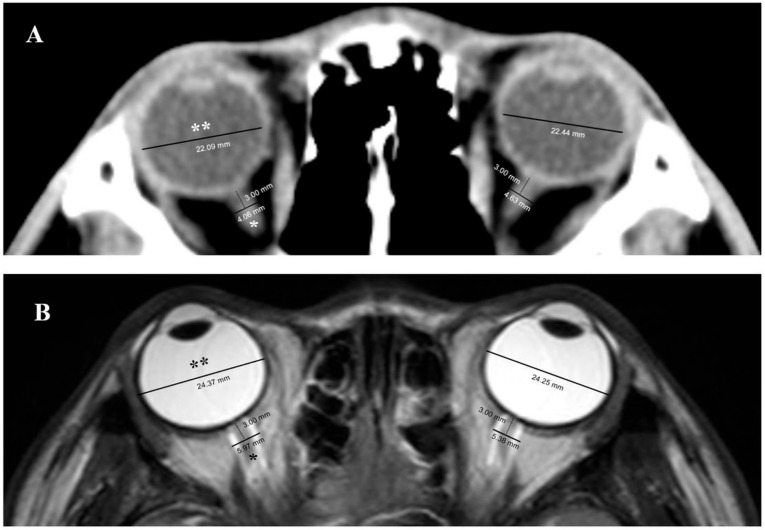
Measurement of the optic nerve sheath diameter and eyeball transverse diameter using computed tomography (A) and magnetic resonance imaging (B). The optic nerve sheath diameter (*) was measured using electronic calipers positioned 3 mm posterior to the globe along a perpendicular vector, while the eyeball transverse diameter () was determined by measuring from the retina to retina.
